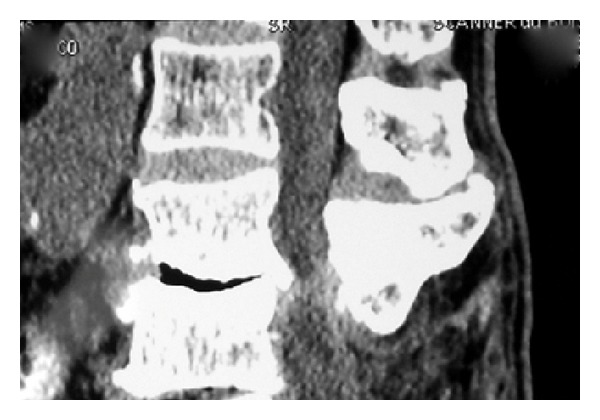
CT scan showing a destruction of L2 vertebral body and L2-L3 disc space. The spinal canal was compromised with paravertebral hypertrophic ossifications (Case 1).
